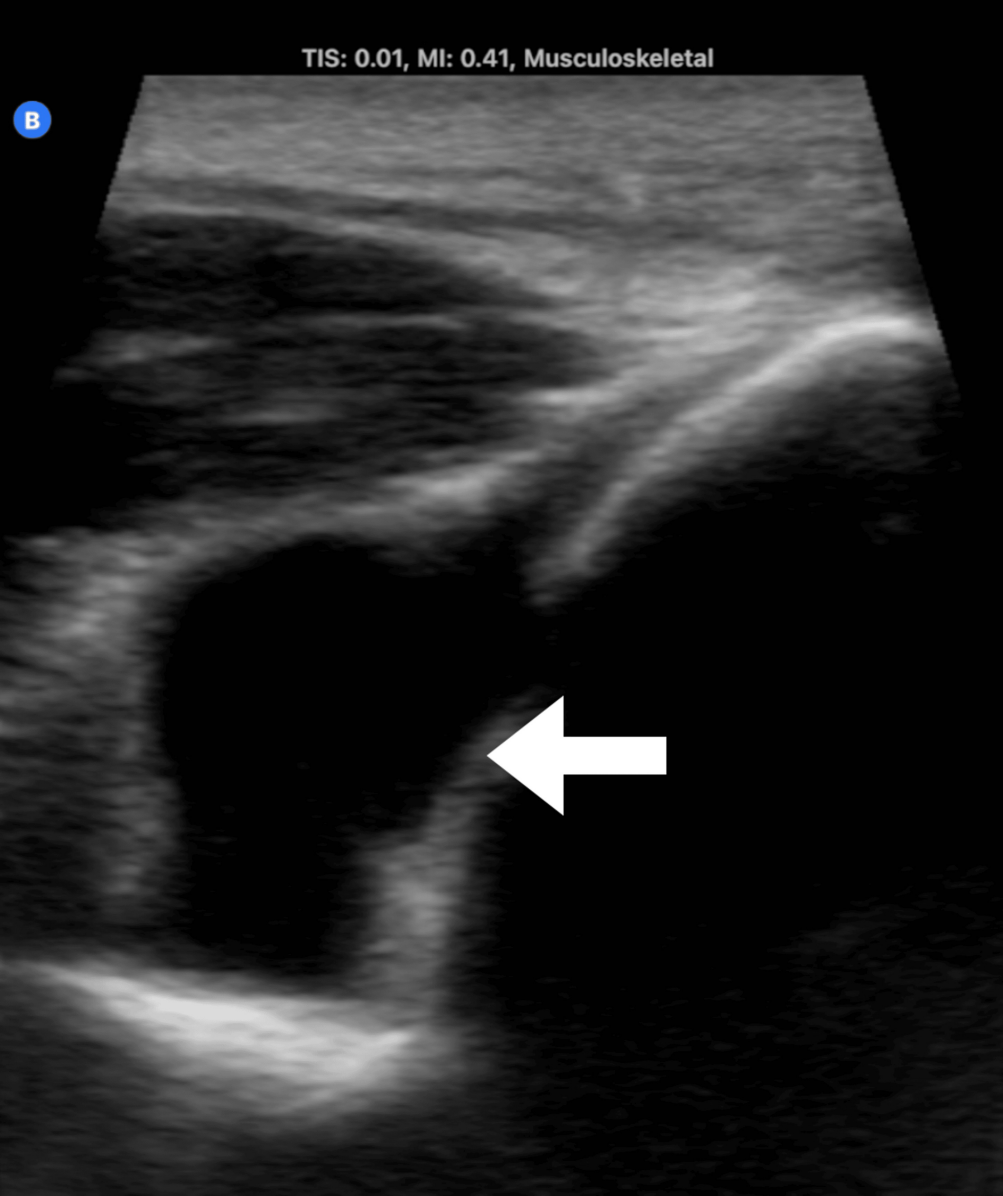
Effusion identified in the left elbow via ultrasound. A longitudinal view of the joint reveals an anechoic fluid collection. The arrow indicates the location of the anechoic effusion in the left elbow joint.
